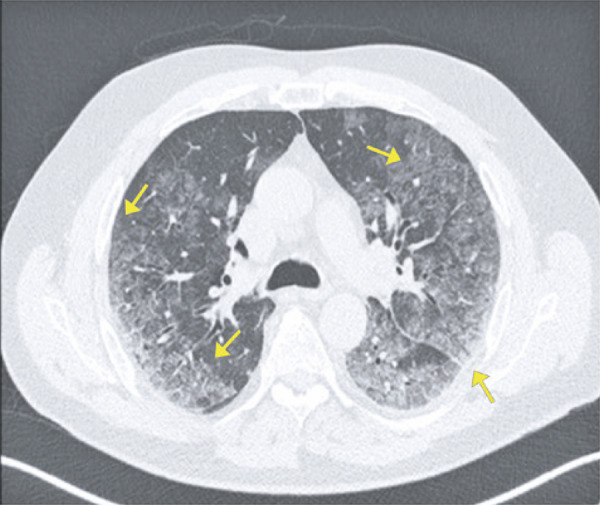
A 38-year-old patient with COVID-19, presenting fever and dry cough for six days. Computed tomography showing diffuse ground-glass opacities associated with thickening of interlobular septa and reticulations (crazy paving)
